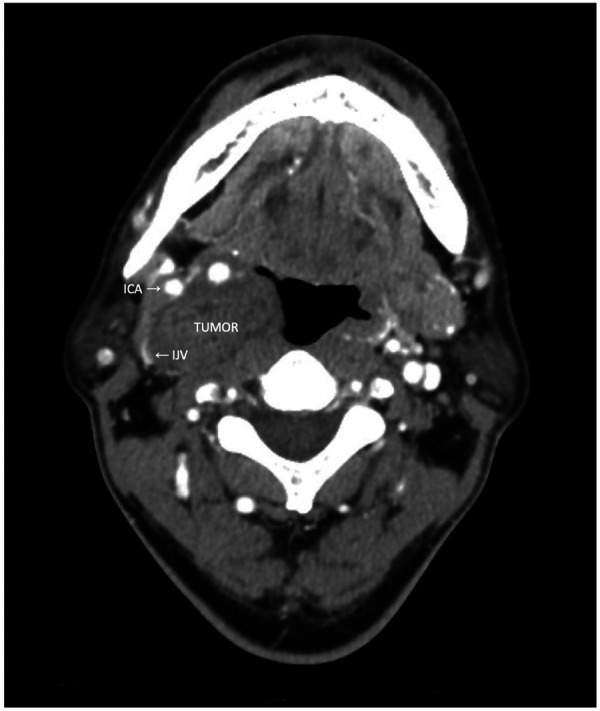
Imaging findings of a vagal schwannoma. Axial contrast-enhanced CT image of the neck shows a well-circumscribed post-styloid parapharyngeal space mass causing separation of the internal carotid artery (ICA) and internal jugular vein (IJV), with anteromedial displacement of the ICA. This vessel displacement pattern was interpreted as suggestive of vagal nerve origin. Intraoperative findings confirmed tumor adherence to the vagus nerve.
